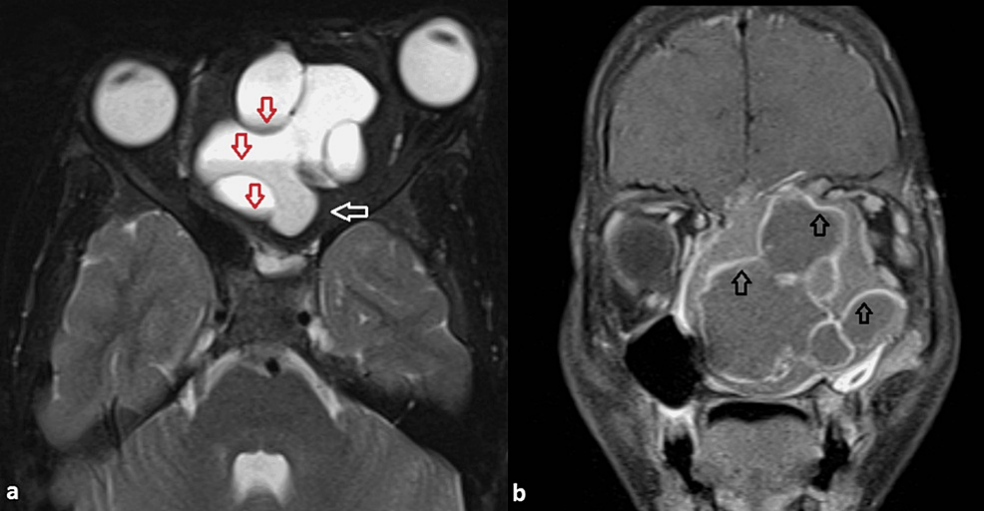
(a) Axial T2 weighted MRI image and (b) Coronal T1 fat-suppressed post-contrast image Fluid-fluid levels are seen within the cystic component of the lesion (indicated by red open arrows in 2a). The periphery of the lesion shows the peripheral T2 hypointense solid component (white open arrow in 2a). Proptosis of the left eye is seen. In the post-contrast image, peripheral enhancement is seen in cystic as well as solid components of the lesion (open black arrows in 2b). MRI - Magnetic Resonance Imaging
